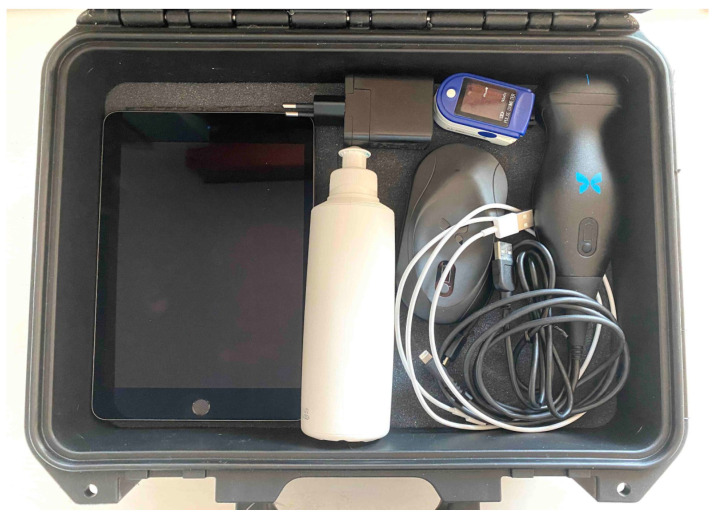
Home sonographic evaluation kit (see the Methods section for details).
